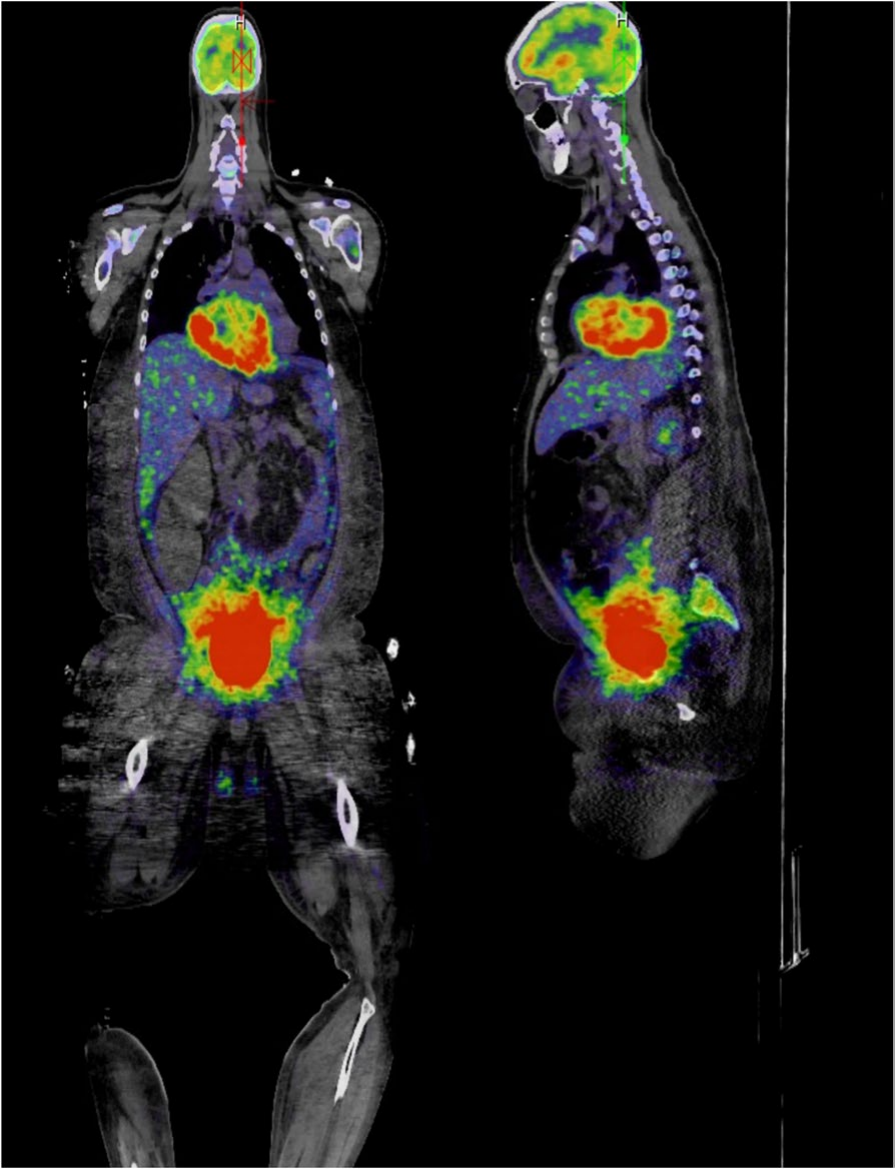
18F-FDG PET/CT scan showing hypermetabolic cardiac mass without alternative primary malignancy
